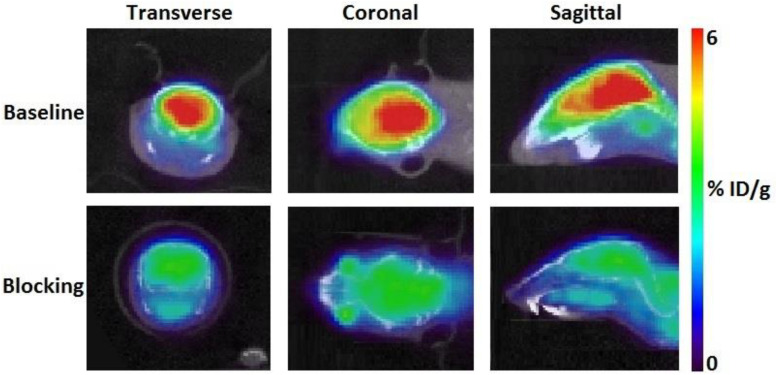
Transverse, coronal and sagittal PET/CT images of [^11^C]iloperidone in baseline (above) and blocking (below) experiments.
